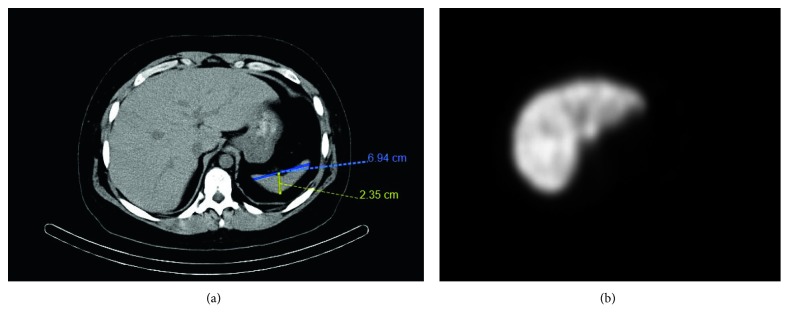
(a) CT showing atrophic spleen without the presence of calcification. (b) Liver-splenic scintigraphy demonstrating hepatic uptake of radioactive ^99m^Tc-labelled colloid but absent splenic uptake.
